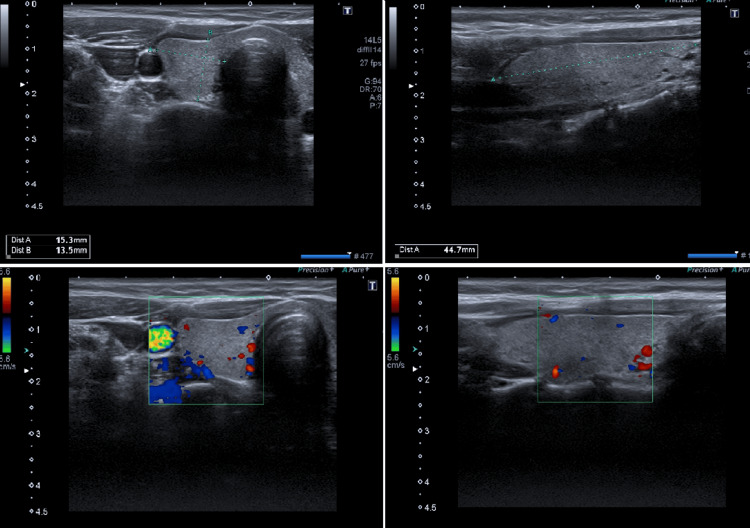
Thyroid ultrasound: thyroid gland with a normal size, homogeneous hyperechoic structure, no apparent nodules, and no increased vascularization on Doppler imaging
